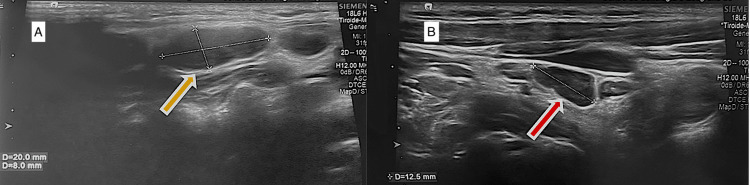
Second ultrasound performed in Primary Healthcare Center Second ultrasound performed in Primary Healthcare Center, revealing the largest hypoechoic ganglion formation (A) and supraclavicular adenopathy (B). Yellow arrow shows the largest ganglion formation measuring 20x8 mm. Red arrow shows the supraclavicular adenopathy measuring 12.5 mm.
